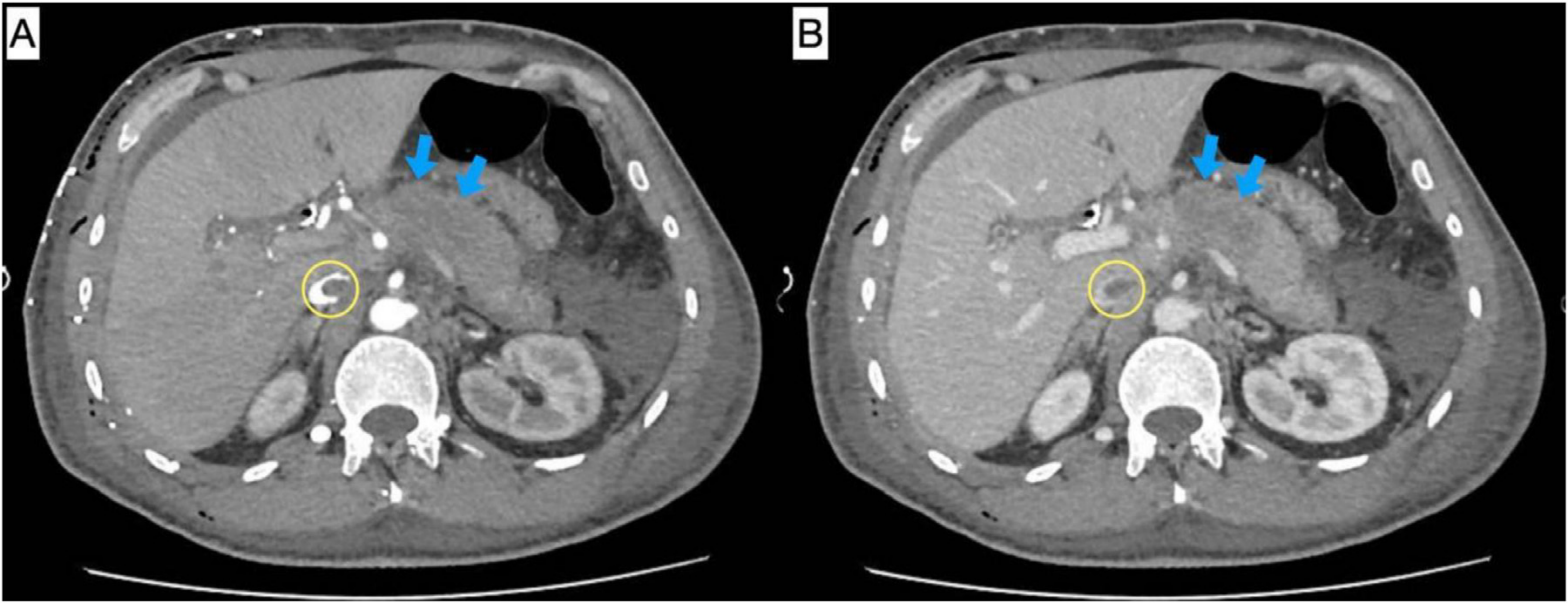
Contrast-enhanced axial CT images in soft tissue window, (A) arterial phase; (B) venous phase. The pancreas (blue arrows), which is heterogeneously enlarged, demonstrates central non-enhancing hypodense areas, consistent with necrosis. Associated findings include peripancreatic fat stranding, upper abdominal free fluid, and regional lymphadenopathy—features suggestive of necrotizing pancreatitis. Yellow circles highlight a filling defect within the inferior vena cava (IVC), suggestive of IVC thrombosis.
